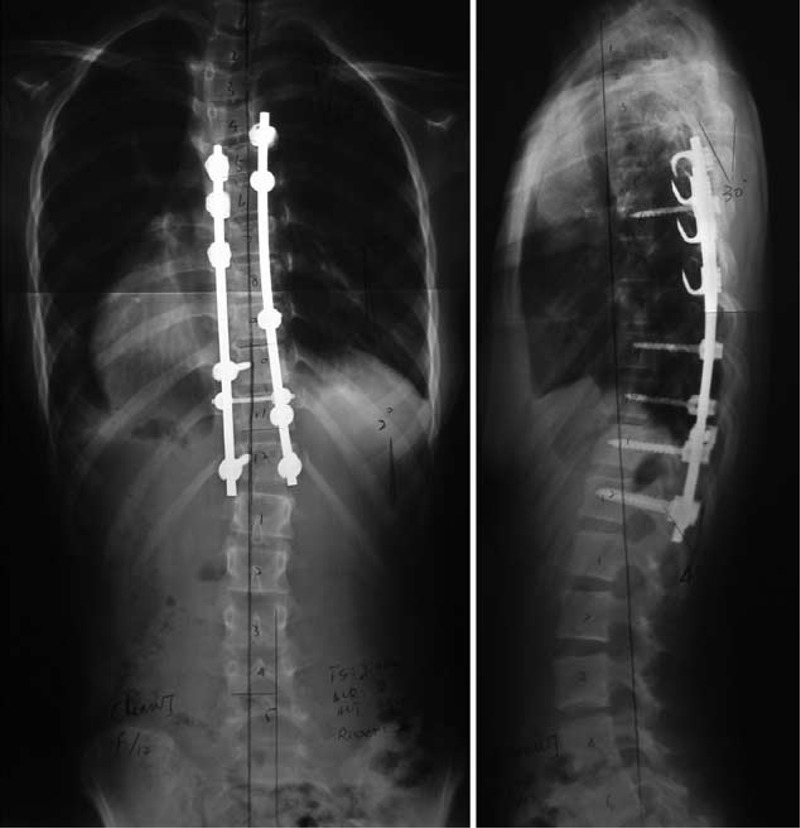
Standing anteroposterior and lateral radiographs of 4 days after operation.
